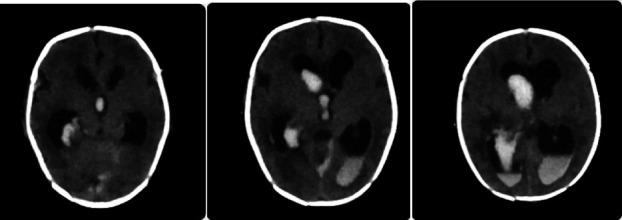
A brain CT scan showing IVH involving the subadjacent parenchyma consistent with Grade IV IVH with mild dilatation of the ventricular system.
